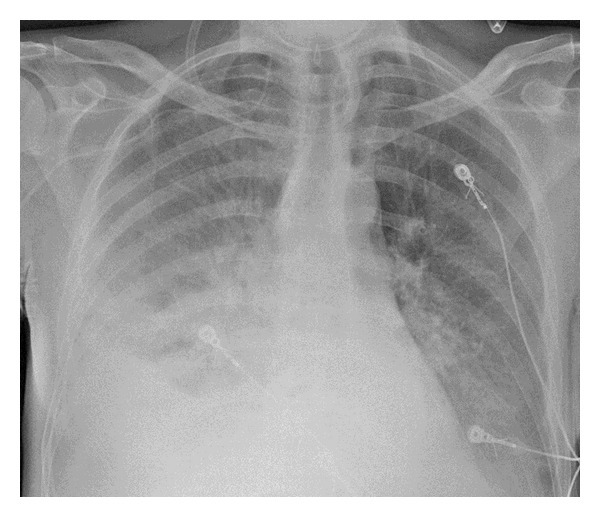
Chest X-ray, 2 h after admission, revealing a rapidly evolving right pleural effusion.
